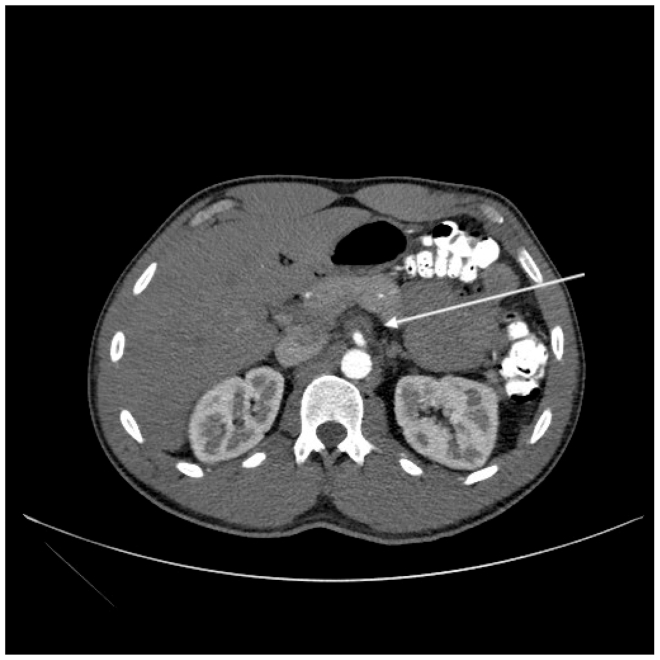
Preoperative computed tomography (CT) scan with intravenous and oral contrast highlighting superior mesenteric artery (SMA) aneurysm with large partially occlusive mural thrombus near take-off from aorta. *Arrow* highlights aneurysmal sac.
